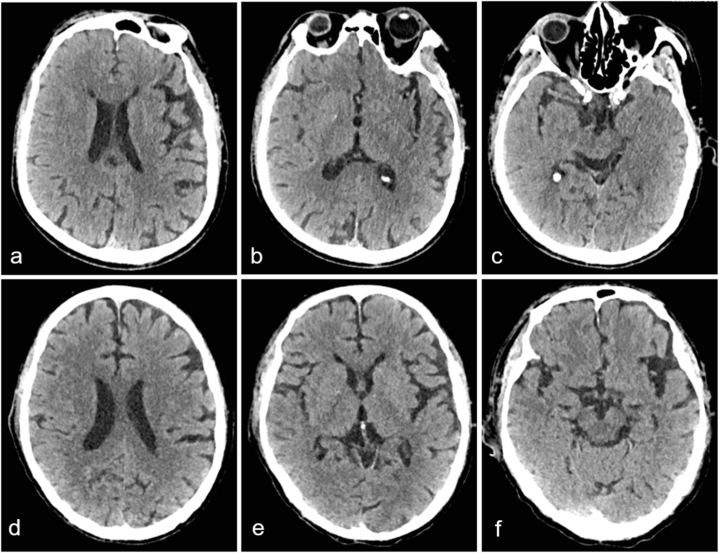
Brain CT scan on day 2 after sintilimab administration and on day 7 after ICU admission. (a–c) The brain CT on day 2 after sintilimab administration. (d–f) The brain CT on day 7 after ICU admission. CT, computed tomography; ICU, intensive care unit.
